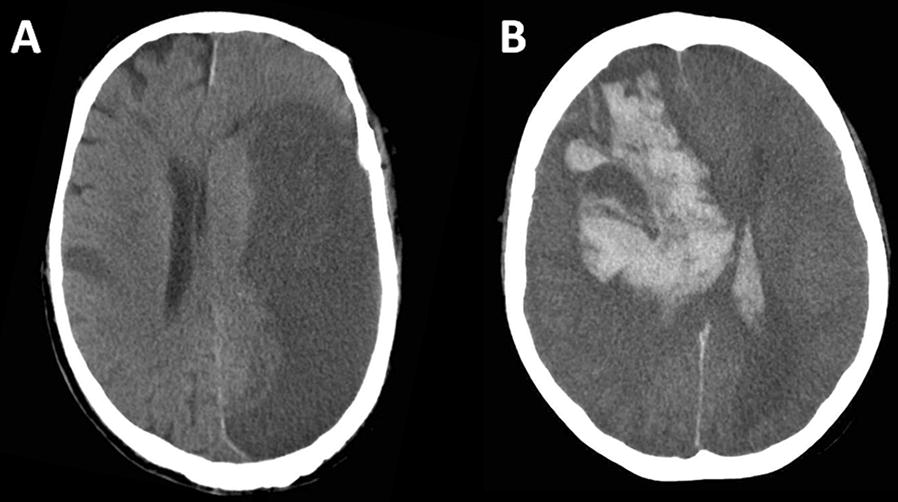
Brain CT-scan showing ECMO-related A massive cerebral infarction and B intraparenchymal hemorrhage
